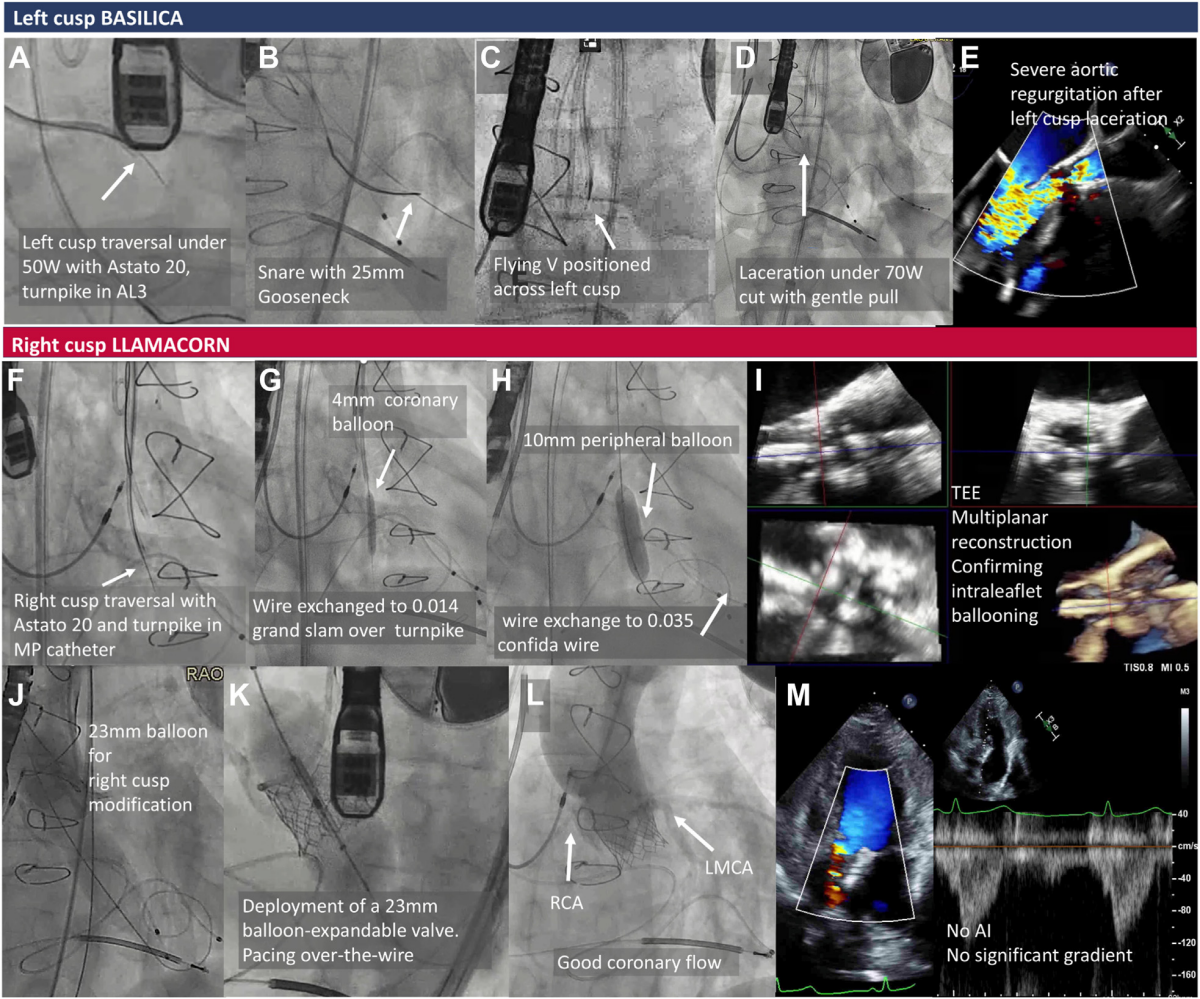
The BASILICA-LLAMACORN hybrid procedure for bileaflet modification. (A-E) Left cusp bioprosthetic or native aortic scallop intentional laceration to prevent iatrogenic coronary artery obstruction during transcatheter aortic valve replacement (BASILICA). (F-K) Right cusp leaflet laceration with balloon mediated annihilation to prevent coronary obstruction with radiofrequency needle (LLAMACORN). (L, M) Results. AI, aortic insufficiency; LMCA, left main coronary artery; RCA, right coronary artery; TEE, transesophageal echocardiogram.
